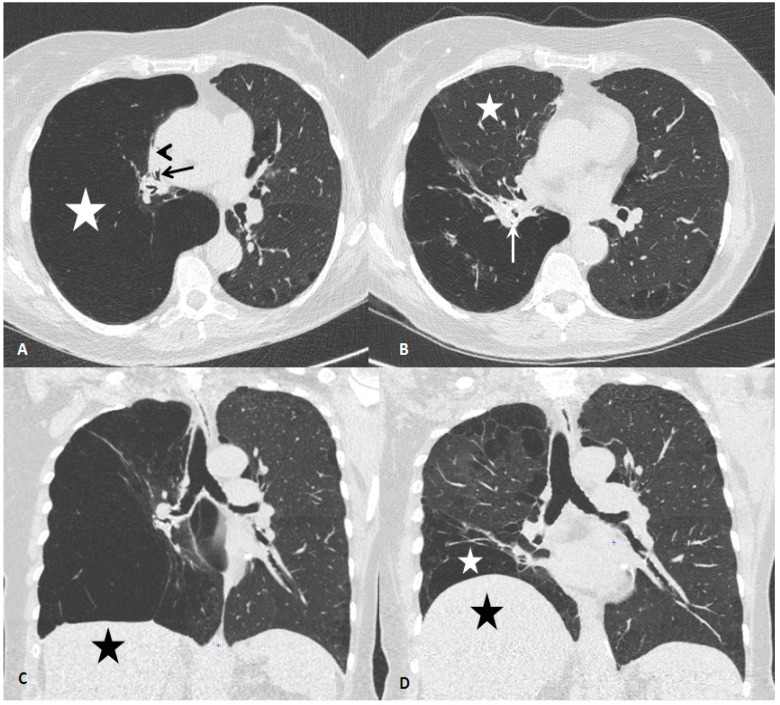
Chest computed tomography before and after endobronchial valves treatment. (A) Axial chest computed tomography (CT) at the level of the right middle bronchus (black arrow), before endobronchial valve treatment, showing complete atelectasis of the right middle lobe (black arrowhead) and extensive emphysema and hyperinflation of the right lower lobe (white star). (B) Axial CT at the same level 1 month after placement of endobronchial valves (EBV), showing right middle lobe re-expansion (white star). One valve is seen in the right lower lobe bronchus (white arrow). (C) CT coronal reconstruction before EBV treatment, showing markedly heterogeneous emphysema and diaphragm flattening (black star). (D) CT coronal reconstruction at the same level 1 month after EBV treatment, showing right lower lobe partial collapse (white star), right hemidiaphragm elevation (black star) and right- and backward mediastinal shift.
